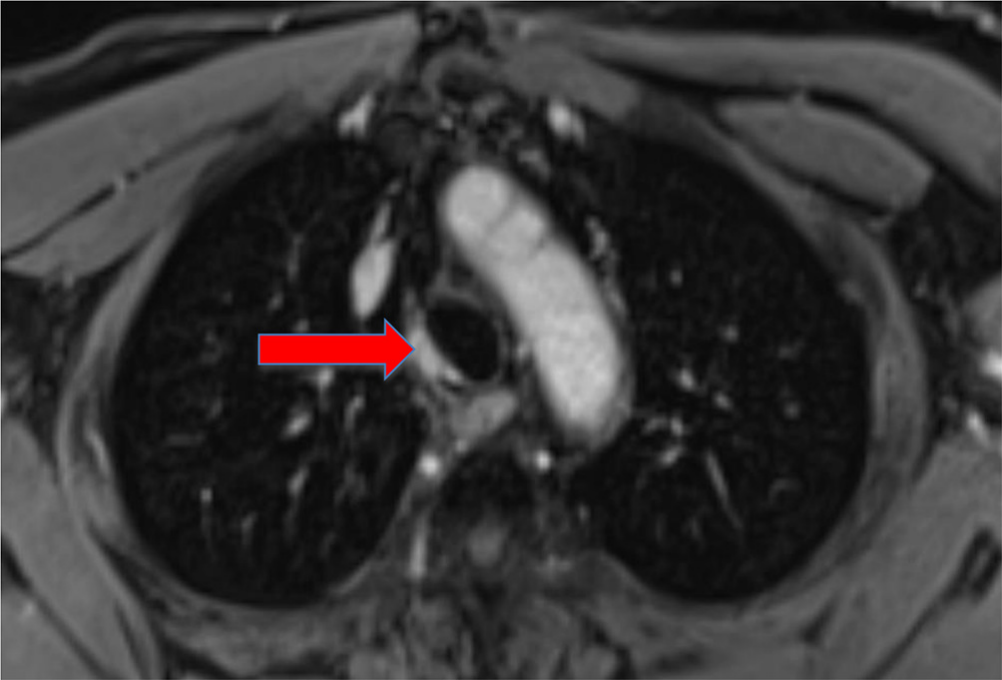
Contrast-enhanced MRI of the mediastinum: axial slice. Block arrow: reduction in tumour size, 1 year post-treatment (November 2012).
